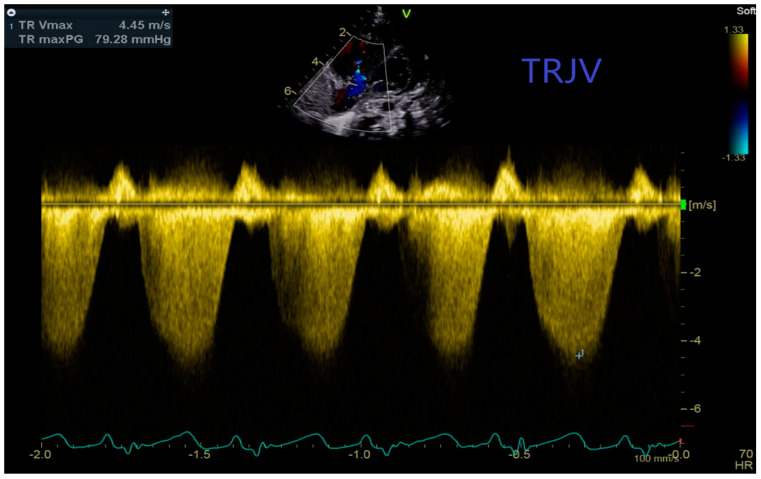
Continuous Doppler trace showing tricuspid regurgitant jet velocity (TRJV) Systolic pulmonary artery pressure estimated using the Bernoulli equation = 84 mmHg [PA pressure = 4 × (TRJV)^2^ + right atrial pressure (5 mmHg)].
